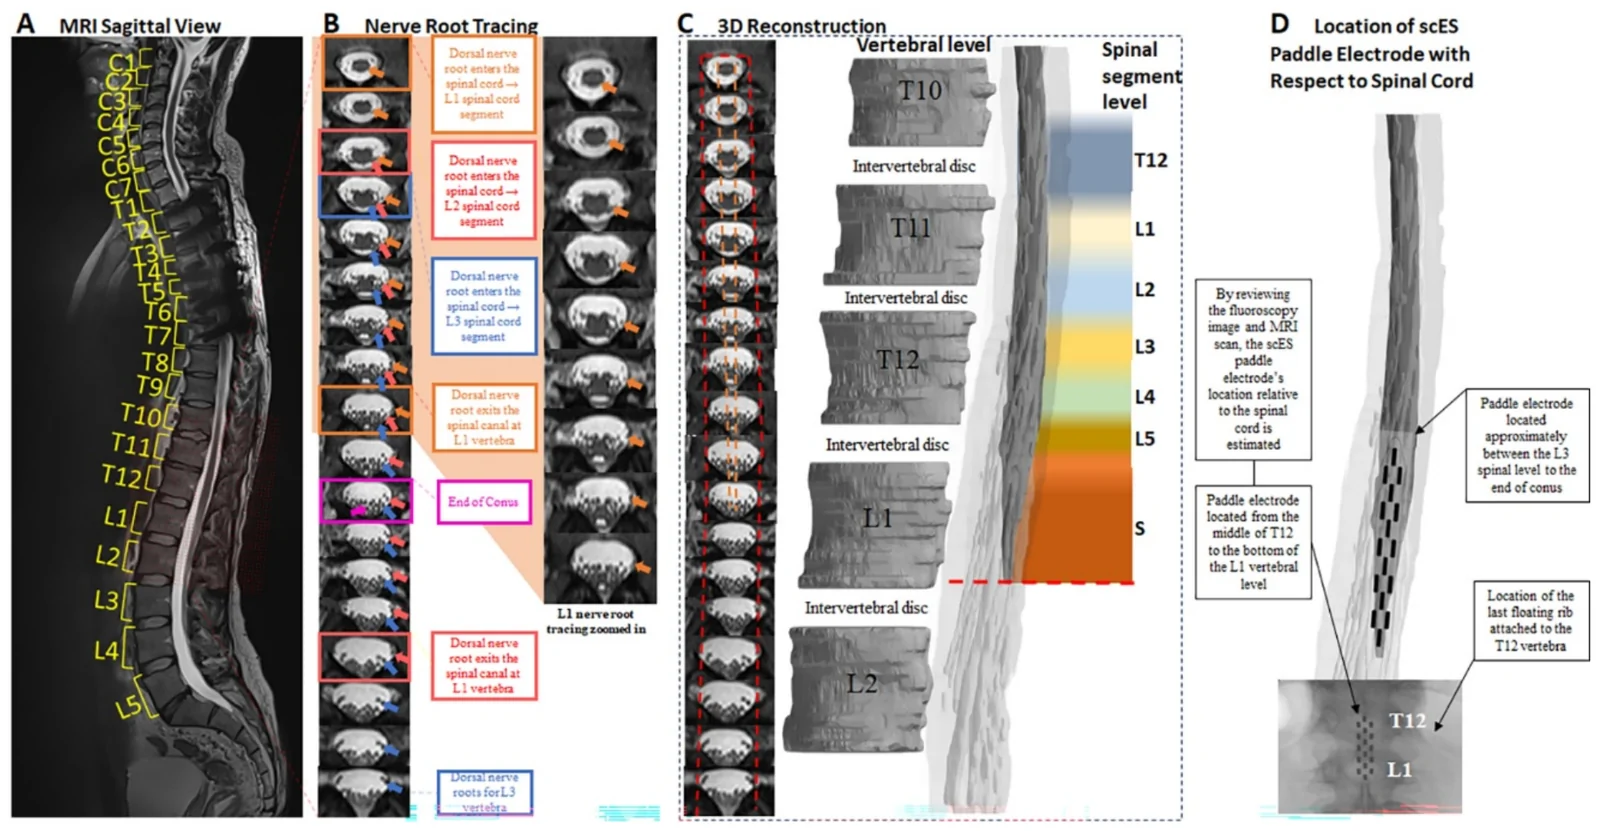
Example of neuroanatomical mapping of the spinal cord at lumbosacral enlargement (Participant ID-A70). (A). MRI sagittal view of the whole spine in an individual with a thoracic spinal cord injury, with the individual vertebral body indicated in yellow. (B). Axial images of the lumbosacral region from a high spatial resolution MRI recording, with the arrows pointing at the dorsal nerve roots from the vertebral site of the exit back to the location of entering the spinal cord to show how spinal cord levels are identified using the nerve root tracing technique. (C). Process of tracing and labeling the spinal cord region (orange dashed lines), cerebrospinal canal (red dashed lines) and nerve roots in each axial image to build a reconstructed model of the lumbosacral spinal cord from the segmented images along with associated vertebral bodies and spinal segment levels. (D). Process of integrating intraoperative fluoroscopy images of the paddle placement with the preoperative MRI recordings to estimate the location of the paddle array on the spinal cord model.
